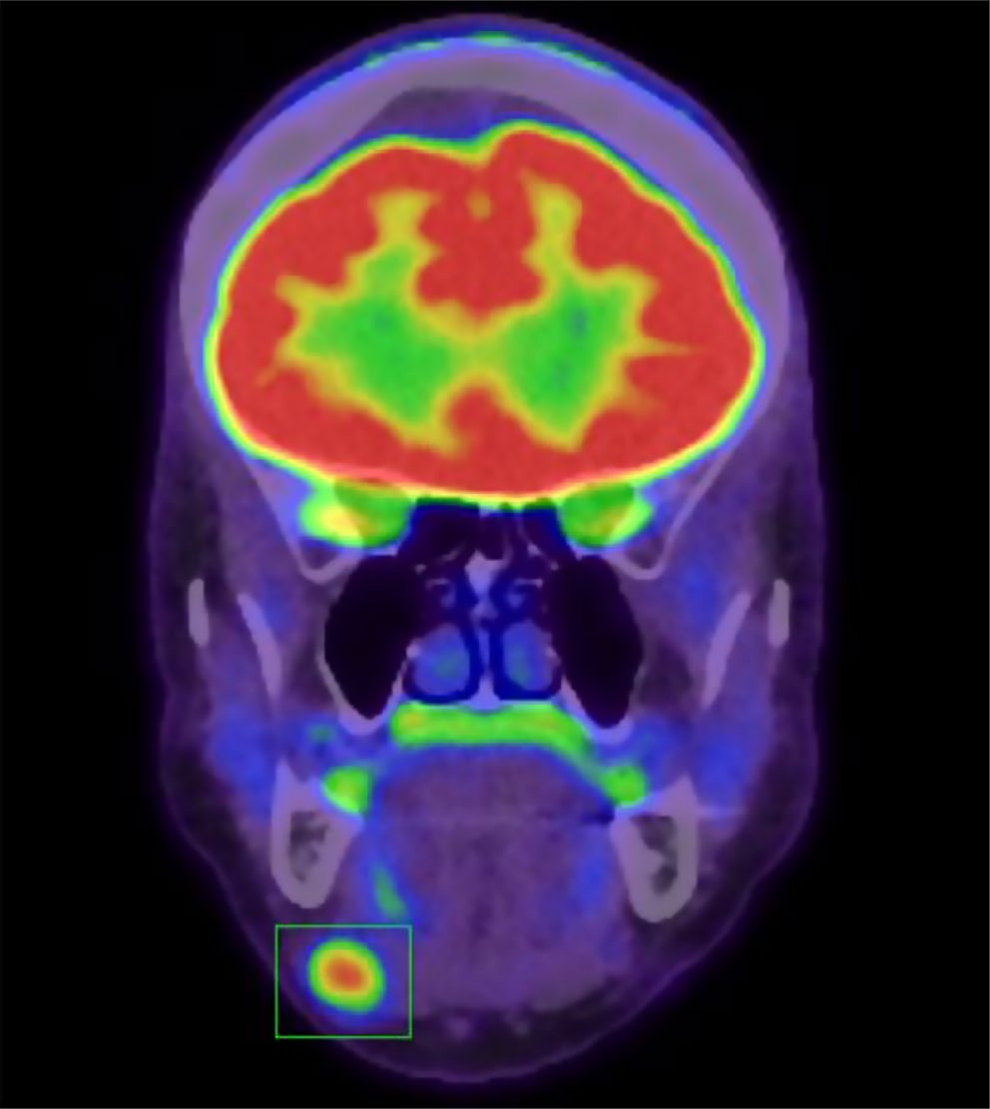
FDG-PET scan demonstrating moderately avid lesion in right submandibular region
